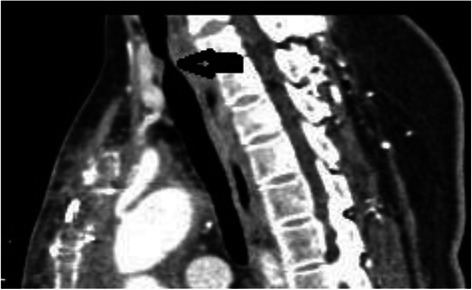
Tracheal stenosis in Case 1: A computed tomographic scan image of a 49-year-old woman (*case 1*) showing severe tracheal stenosis, with diameter of 3.5 mm for a length of over 1 cm near the region of the thyroid isthmus (*arrow*)
